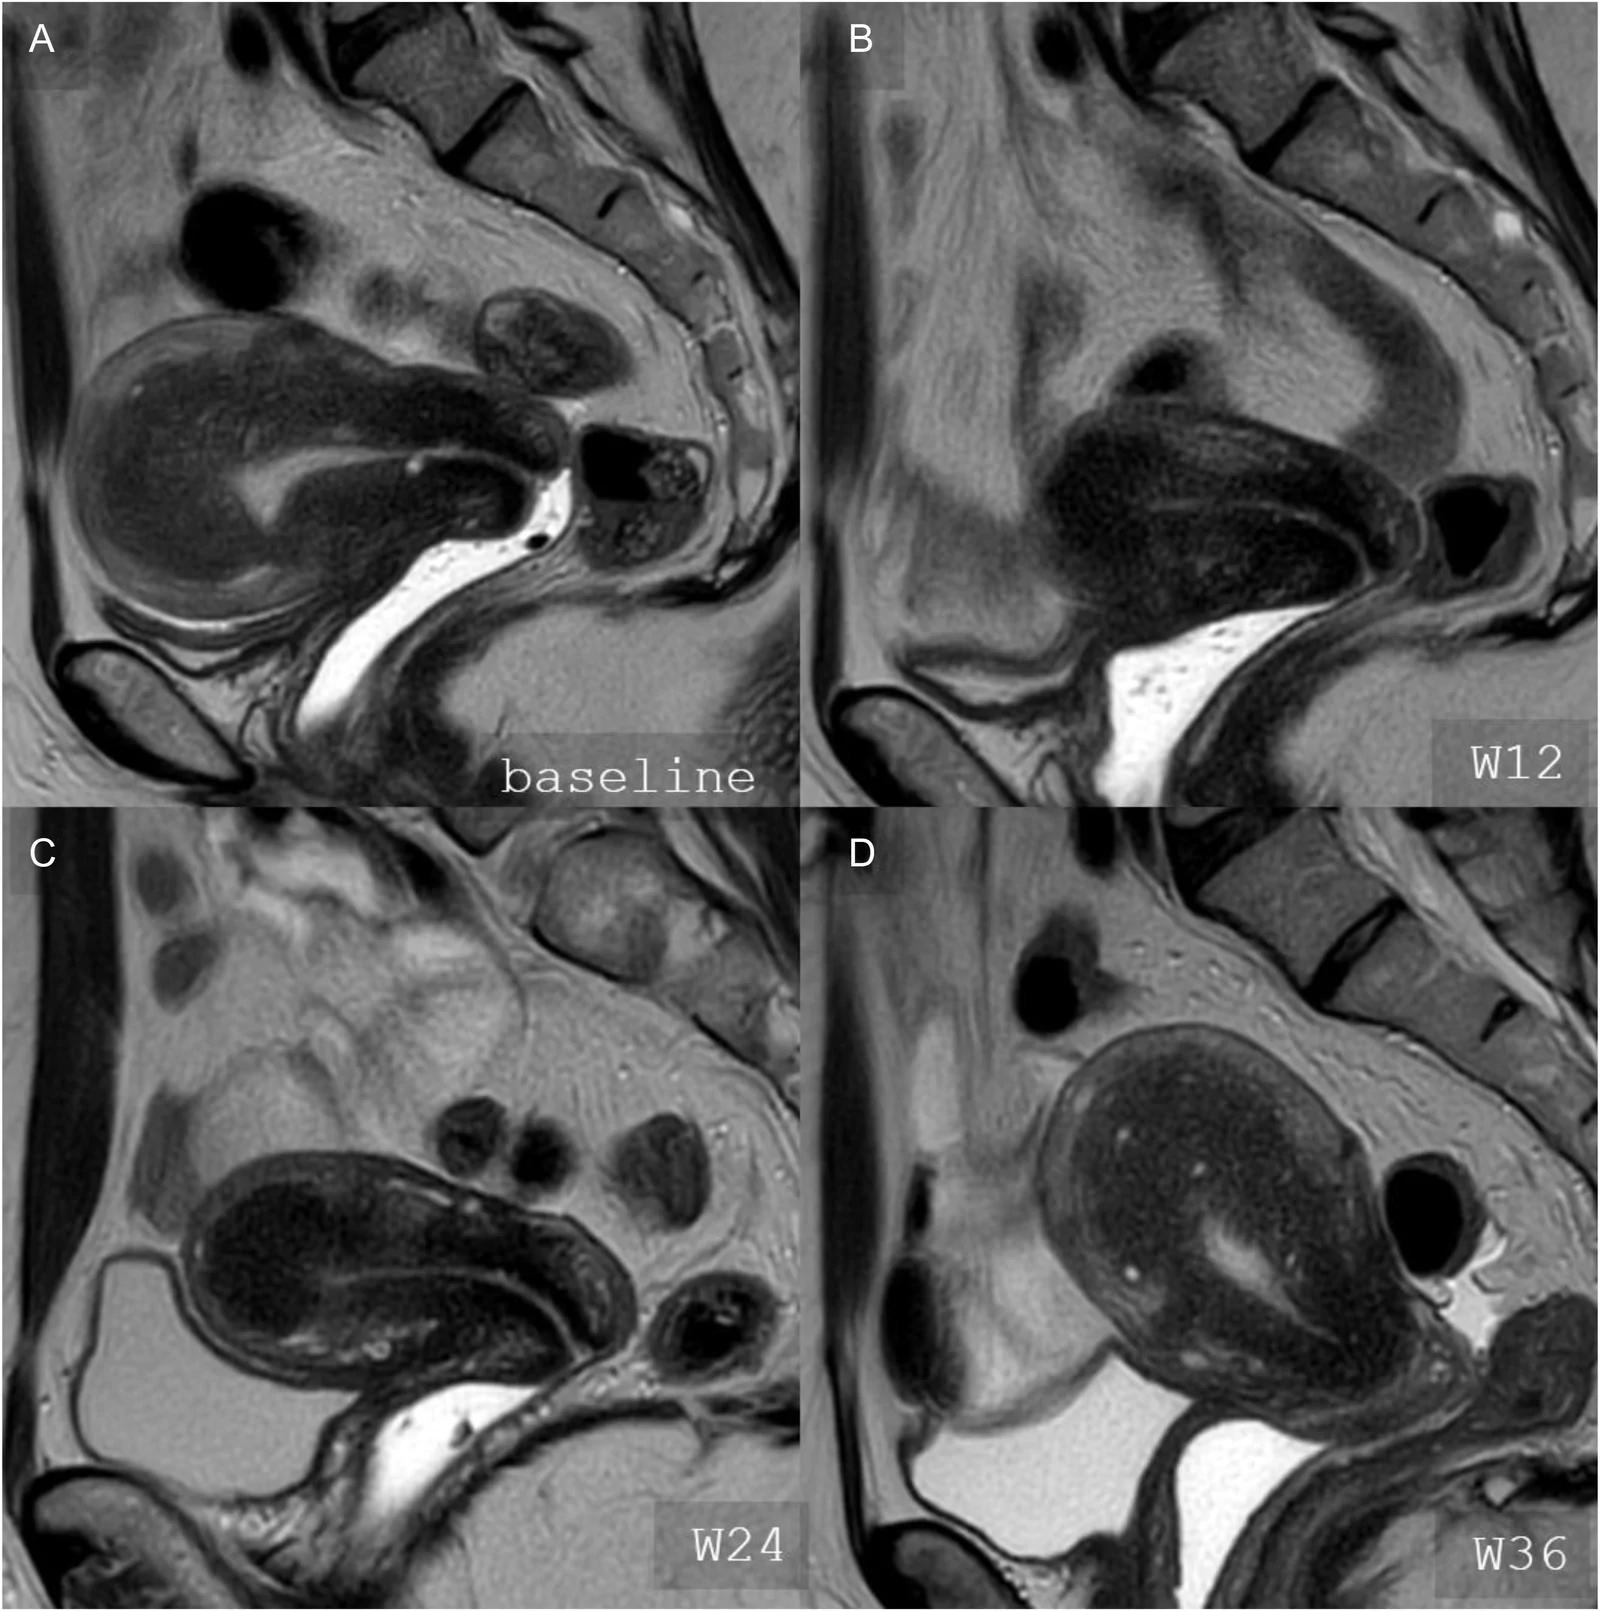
Patient 2. (A) MRI showing an enlarged uterus with diffuse and disseminated adenomyosis at baseline. (B) After 12 weeks of GnRH antagonist therapy (200 mg linzagolix, QD), a significant reduction was observed in uterine size and adenomyotic lesions. (C) The patient was then switched to 100 mg/day linzagolix, causing some regrowth, but the volume remained significantly smaller than at baseline. (D) By week 36 (12 weeks after the end of therapy), uterine volume values returned to baseline values.
